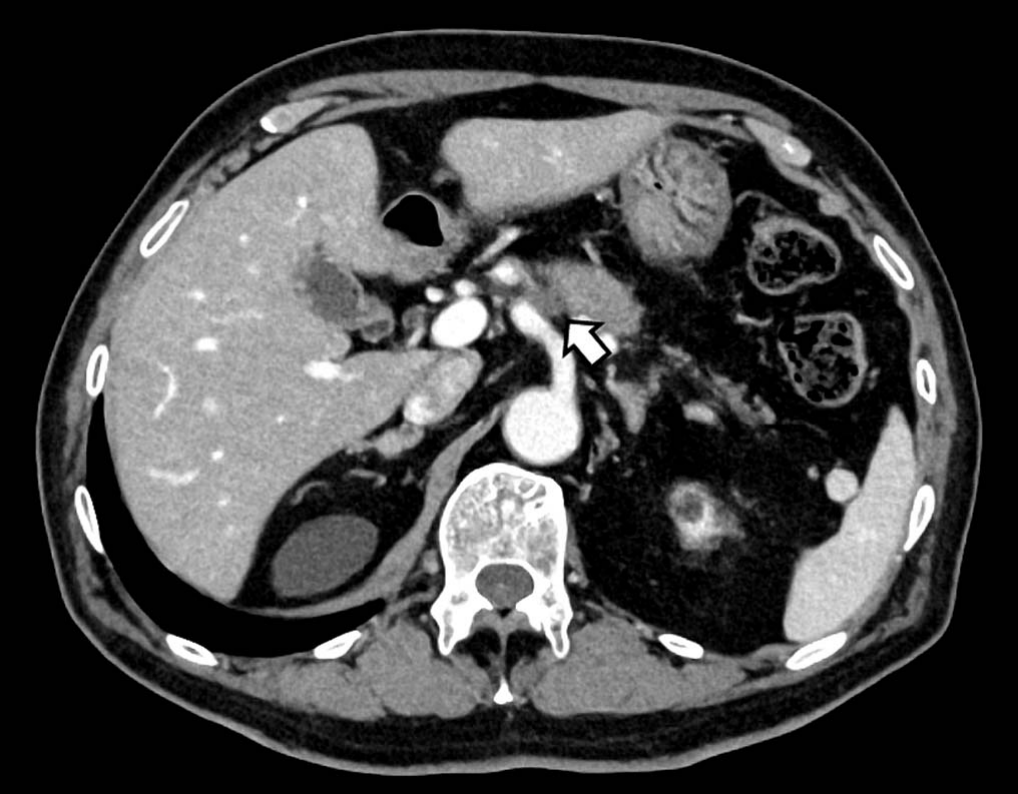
Computed tomography after preoperative chemotherapy showing tumor invasion on the left side of the celiac artery.
